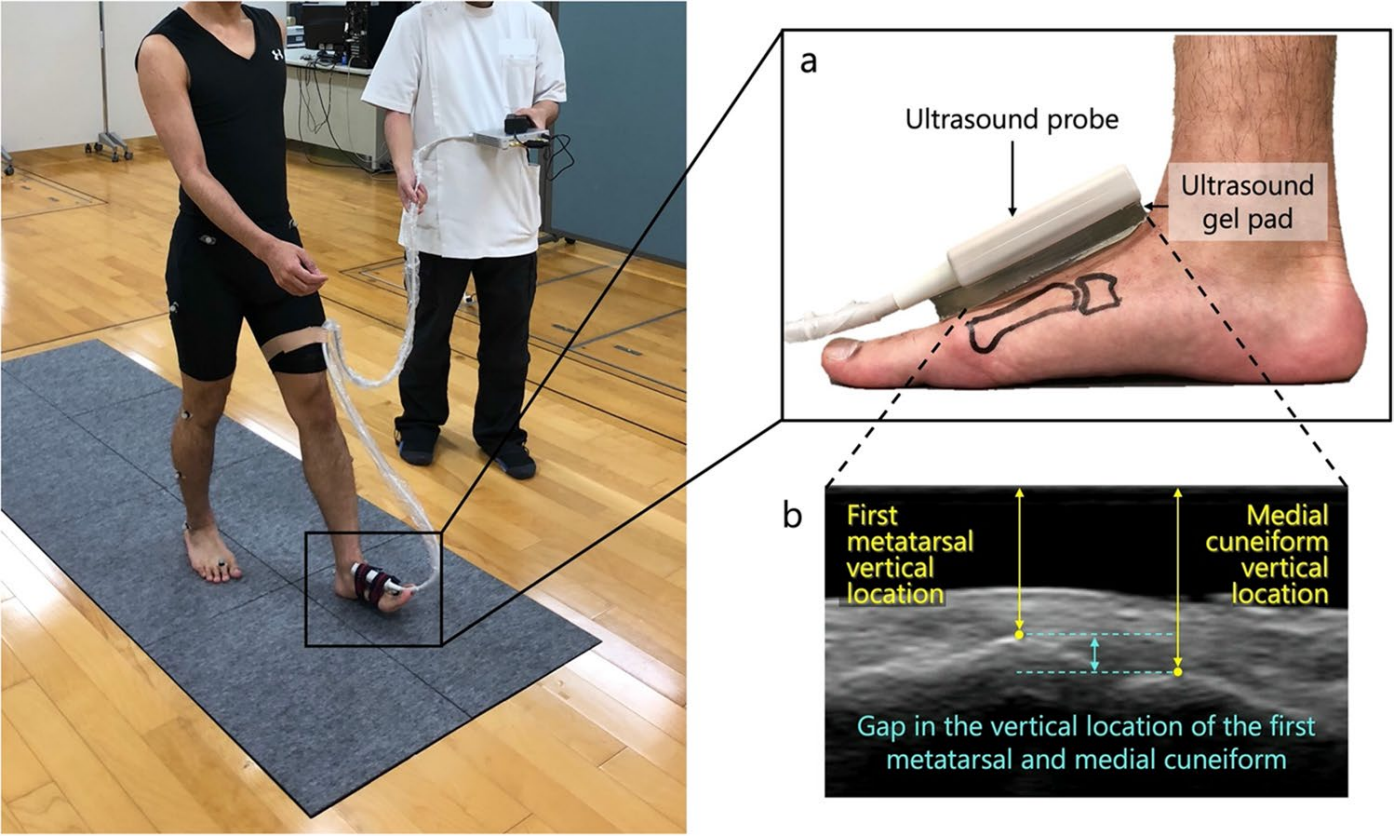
Gait analysis using ultrasound and a three-dimensional motion analysis system. Ultrasound probe was attached at the tarsometatarsal joint on an ultrasound gel pad (a), and the first metatarsal and medial cuneiform were within the field of view (b). The vertical locations of the first metatarsal and medial cuneiform were defined as the vertical distance from the screen top to the dorsum of each bone.
